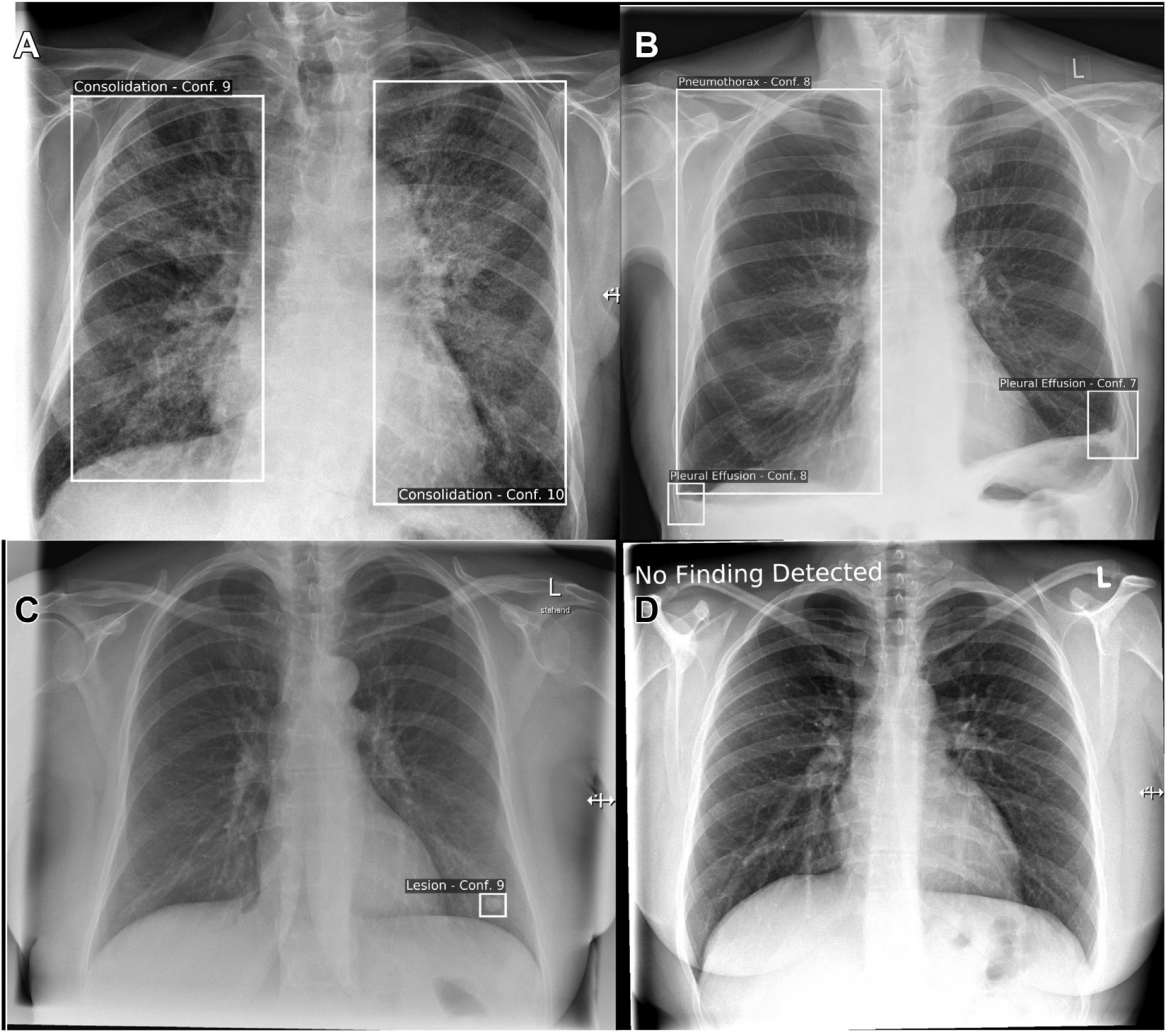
A-D, Examples of artificial intelligence secondary captures. A, Correctly identified bilateral consolidations that are suspicious for pneumonia considering the depth of inspiration. B, Both the right-sided seropneumothorax with basal air-fluid level as well as the shadowing in the left costodiaphragmatic recess (possible pleural effusion or pleural fibrosis) were identified. C, The solitary nodule in the left lower lung was correctly identified. D, In case the artificial intelligence algorithm did not identify any of the four pathologies on the chest radiograph, the image was marked as “No Finding Detected.”
